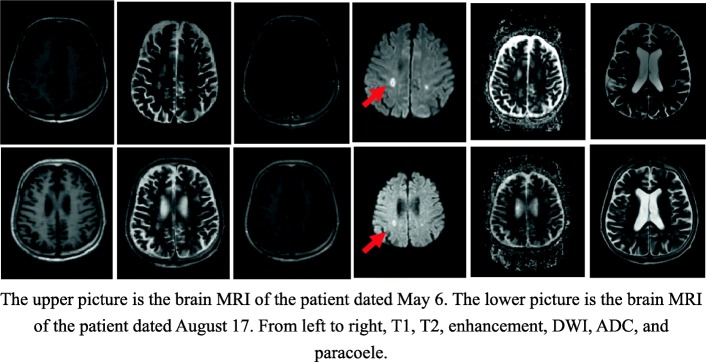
CNS MRI before treatment (above) and after treatment (below). The upper picture is the brain MRI of the patient dated May 6. The lower picture is the brain MRI of the patient dated August 17. From left to right, T1, T2, enhancement, DWI, ADC, and paracoele
